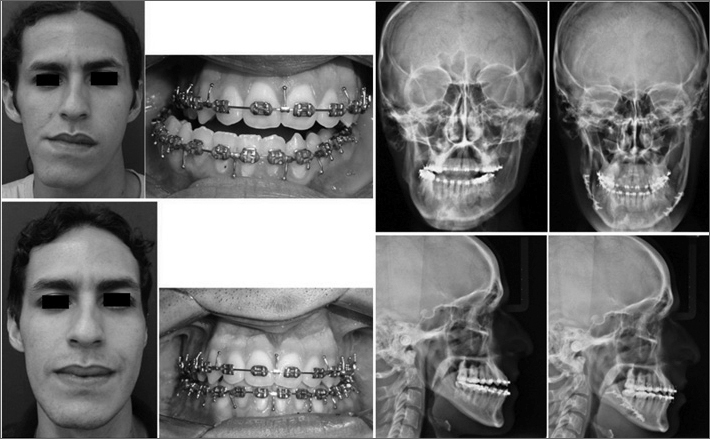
Patient pictures and X-ray images before and after surgery.
